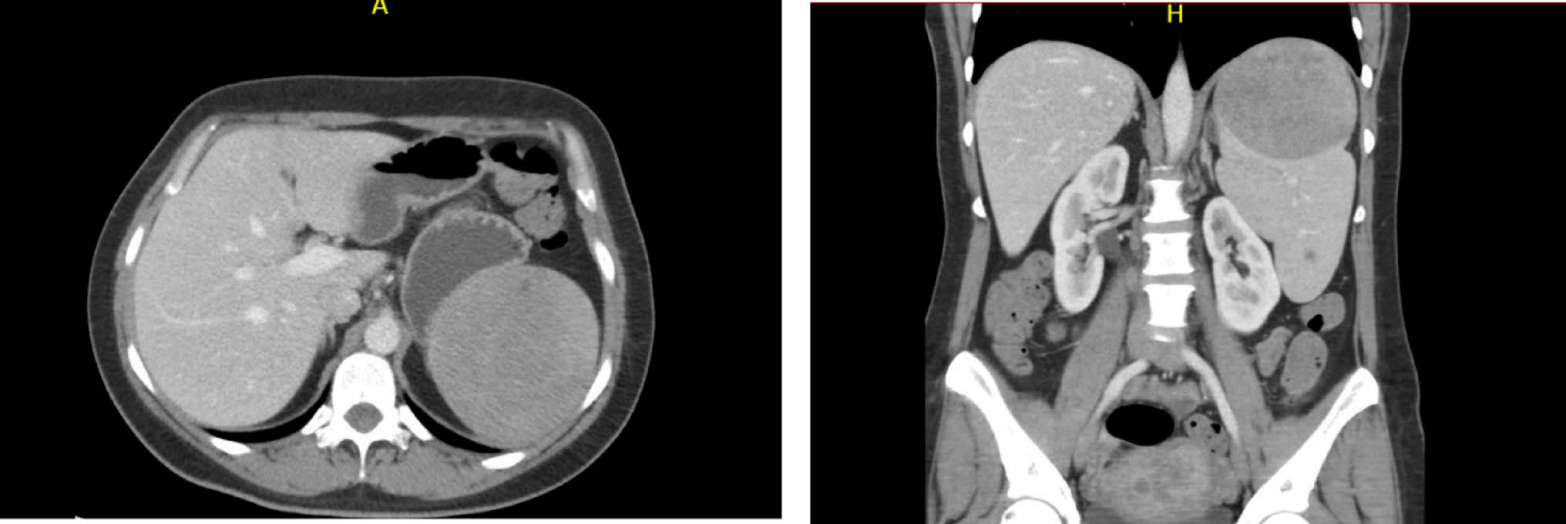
CT scan of the patient showing the splenic hamartoma above the upper pole of the left kidney in coronal (left) and axial (right) planes
